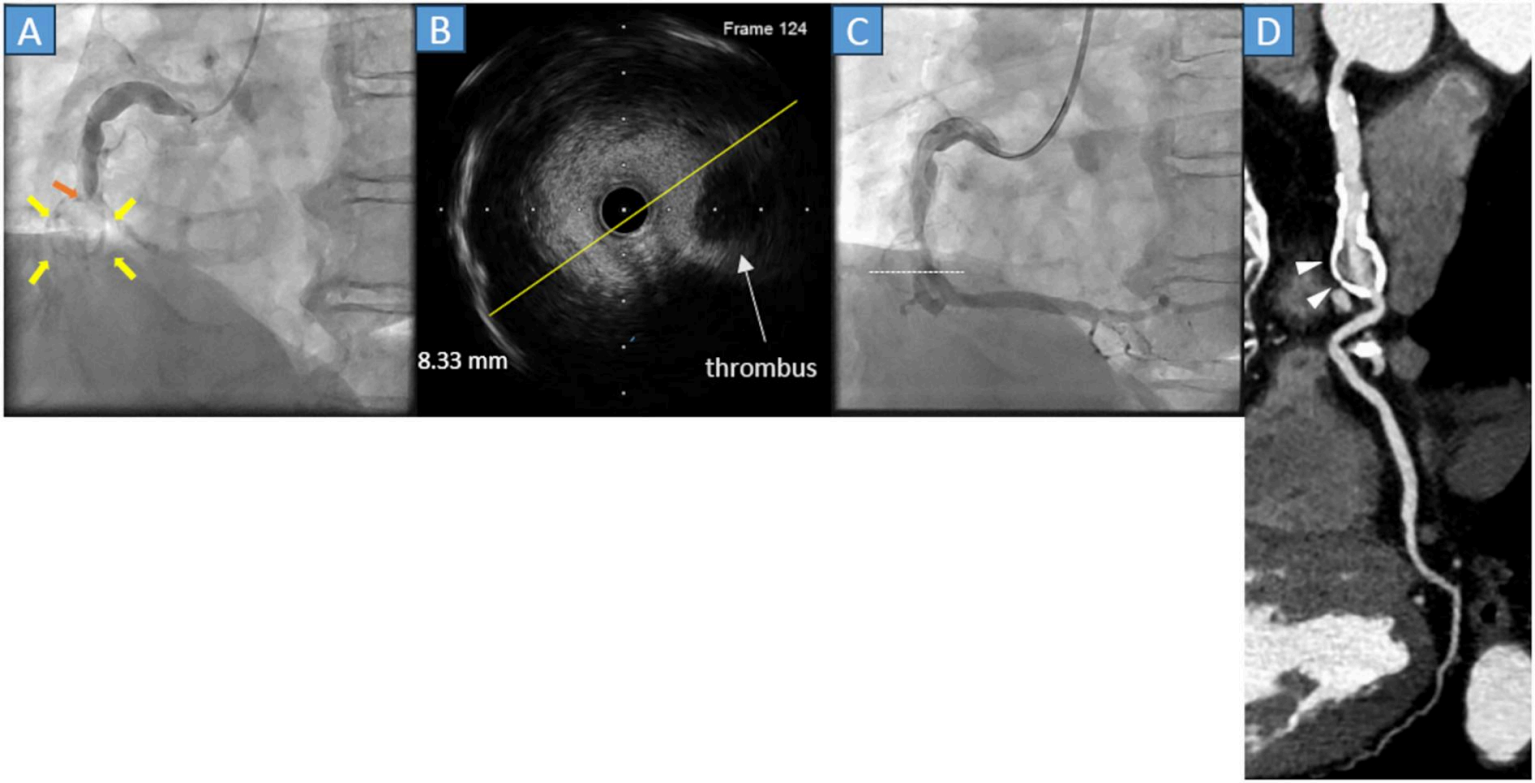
(A) Coronary angiography (CAG) reveals acute total occlusion of the middle right coronary artery (orange arrow) with a circular calcified structure (yellow arrows). (B) The cross-sectional image of the intravascular ultrasound (IVUS) reveals that the diameter of the lesion was 8.33 mm (yellow line) with thrombus and calcification of the vessel wall. (C) The final CAG reveals a Thrombolysis in Myocardial Infarction grade III flow. The white dots in the angiography correspond to the cross-sectional IVUS image. (D) Cardiac computed tomography in the chronic phase reveals that preserved right coronary flow and the presence of a saccular aneurysm with severe calcification (gray arrowheads).
